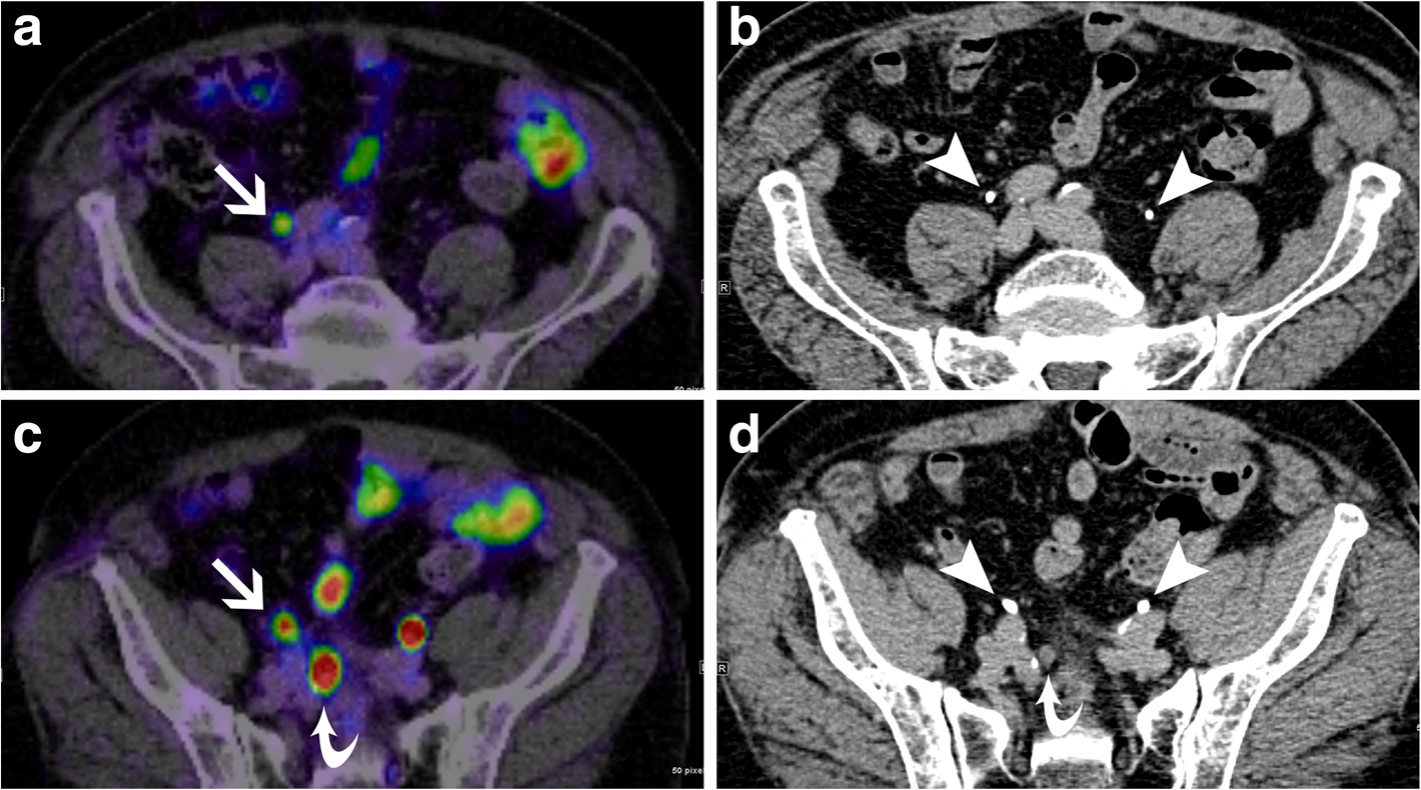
Discrepancies for ^68^Ga-PSMA-11 PET/CT without and with CTU in anatomical assignment of tracer accumulation in a patient with multifocal retroperitoneal nodal relapse. a shows focal tracer accumulation in the right iliac retroperitoneum that was assigned to tumor uptake in ^68^Ga-PSMA-11 PET/CT alone (arrow in a, confidence score 1) and to right ureteric excretion in ^68^Ga-PSMA-11 PET/CT with CTU (arrow heads in b refer to both ureters, confidence score 3). c again shows focal tracer accumulation in the right iliac retroperitoneum that was assigned to tumor uptake in ^68^Ga-PSMA-11 PET/CT alone (arrow in c, confidence score 1) and to right ureteric excretion in ^68^Ga-PSMA-11 PET/CT with CTU (arrow heads in b refer to both ureters, confidence score 3). There is another focal tracer accumulation that was assigned to metastatic disease in ^68^Ga-PSMA-11 PET/CT without and with CTU (curved arrow in c and d, both confidence score 3)
